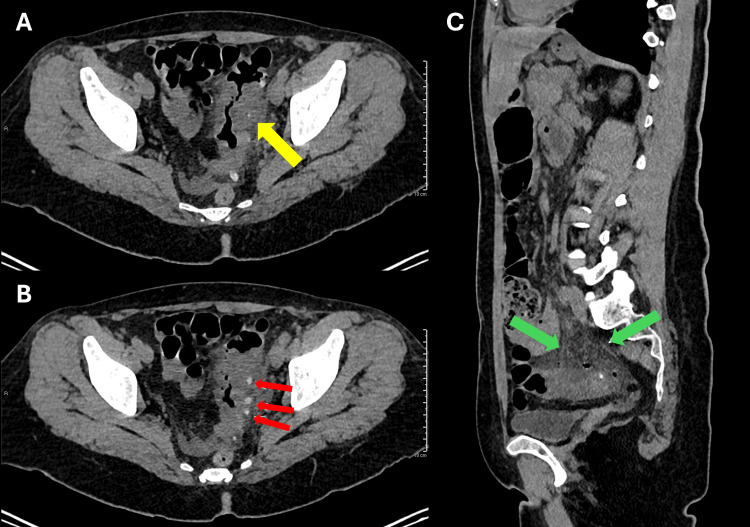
Non-contrast abdominal CT findings consistent with acute sigmoid diverticulitis. (A, B) Axial images showing parietal thickening of a sigmoid diverticulum (yellow and red arrows) and adjacent wall. (C) Sagittal image demonstrating densification of the adjacent mesenteric fat (green arrows).
